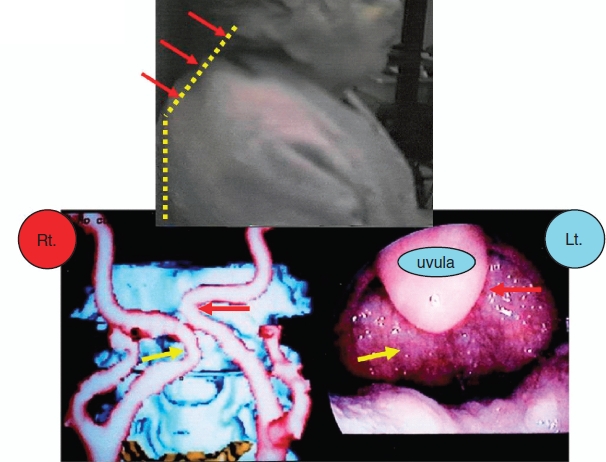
Top: actual bent-posture in an 84-year-old female. (yellow dots). Lower: three-dimensional computed tomography (CT) showing aberration of the internal carotid artery on both sides. Aberrant carotid arteries in the mouth. A submucosal swelling pulsates in synchrony with the patient's heartbeat. Aberration of the internal carotid artery. Reproduced with permission from Tsunoda K et al. Ann Intern Med 2003; 139:W-56 [8].
